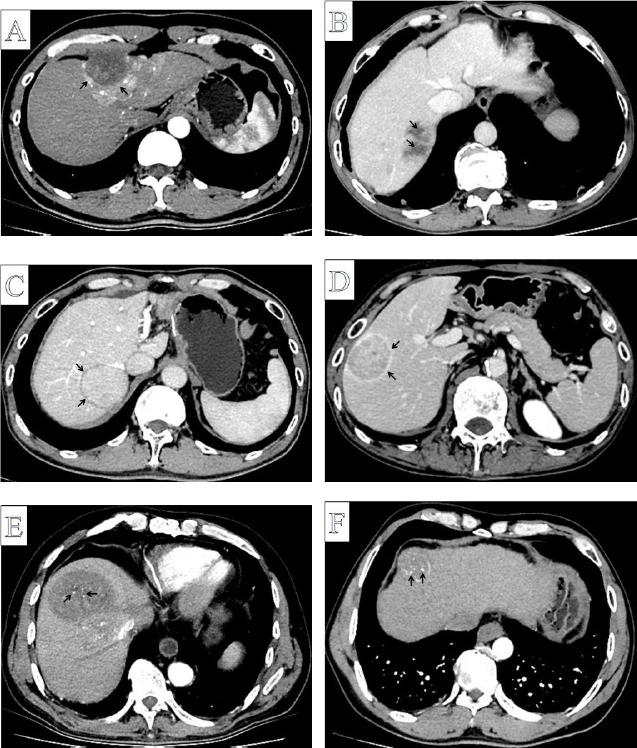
Enhanced CT imaging features. (A) Peritumoral hepatic parenchymal enhancement (arrow). (B) Intratumoral necrosis (arrow). (C) Absence of enhancing capsule (arrow). (D) Enhancing/well-defined capsule (arrow). (E, F) Intratumoral arteries (arrow).
